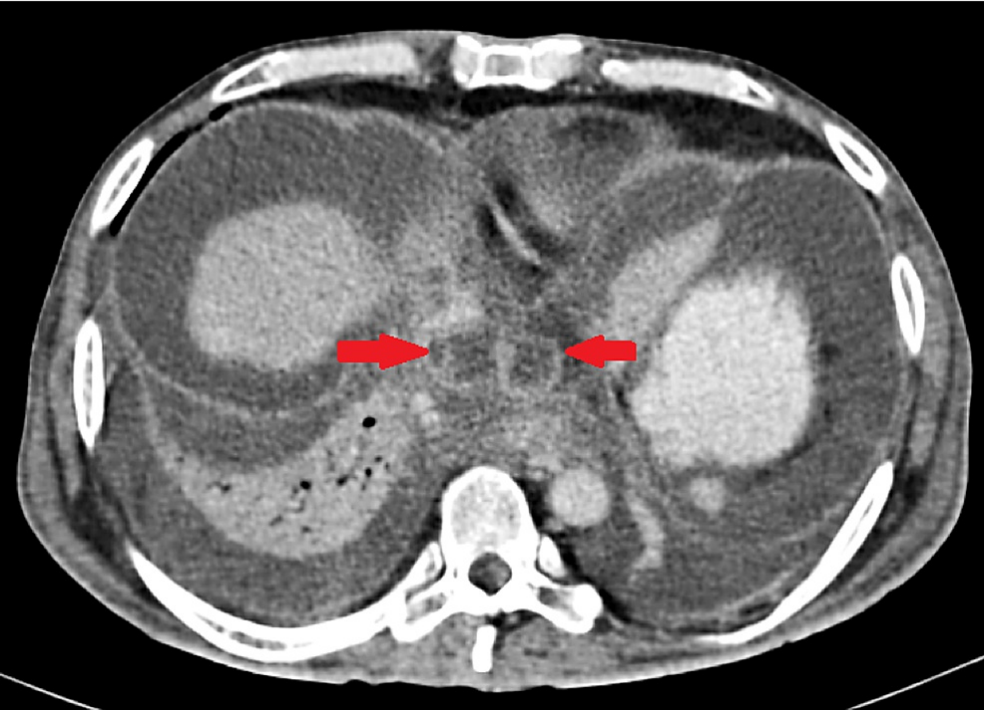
Contrast-enhanced computed tomography of the abdomen showing pseudocyst at diaphragm level, traversing the hiatus
